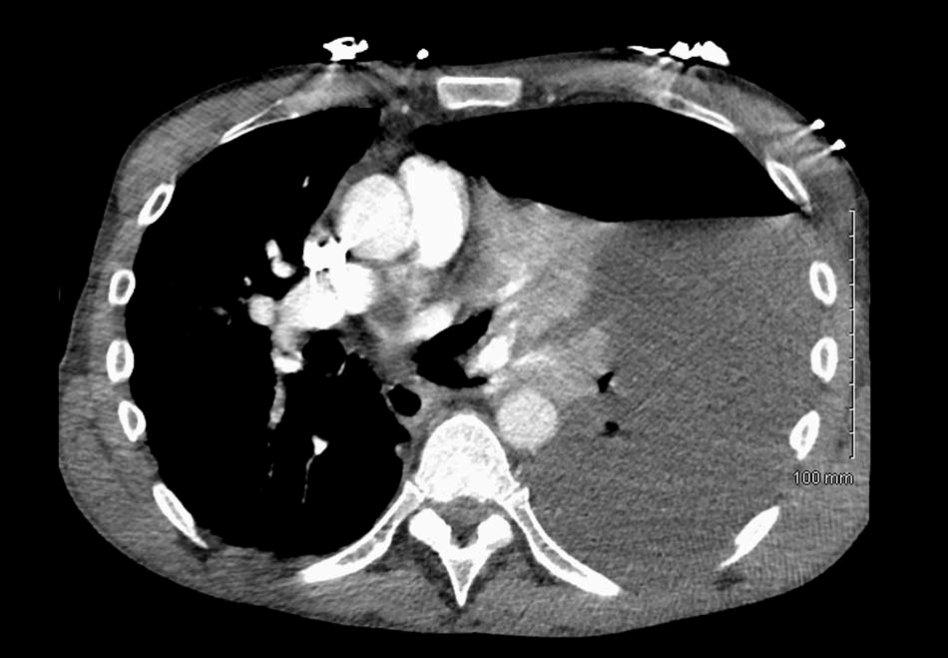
Massive haemopneumothorax on CT.
